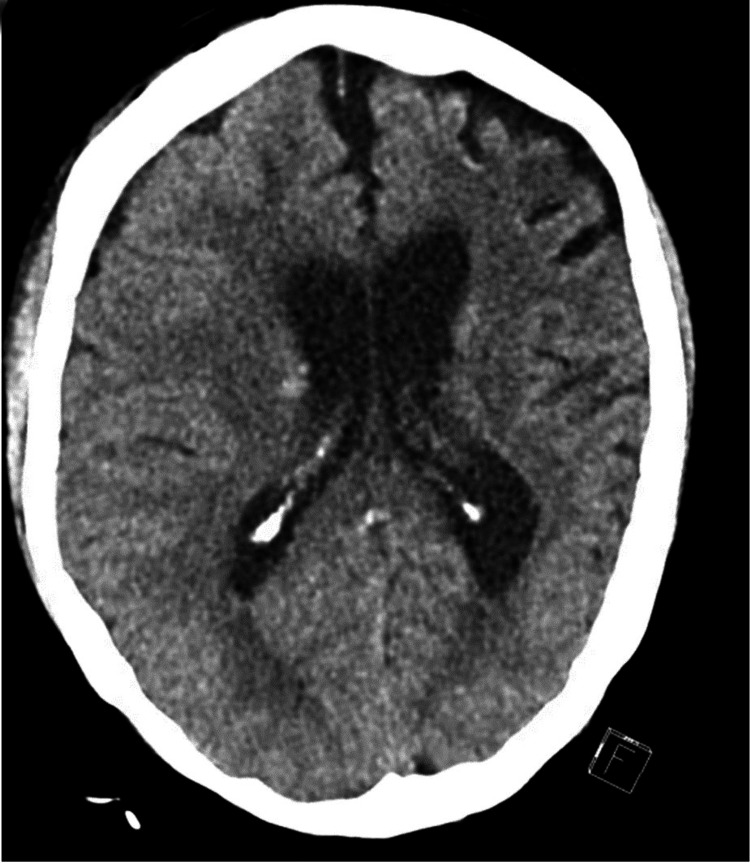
Repeat CT head after EVD drain removal with slight improvement of midline shift and edema CT: computed tomography, EVD: external ventricular drain
